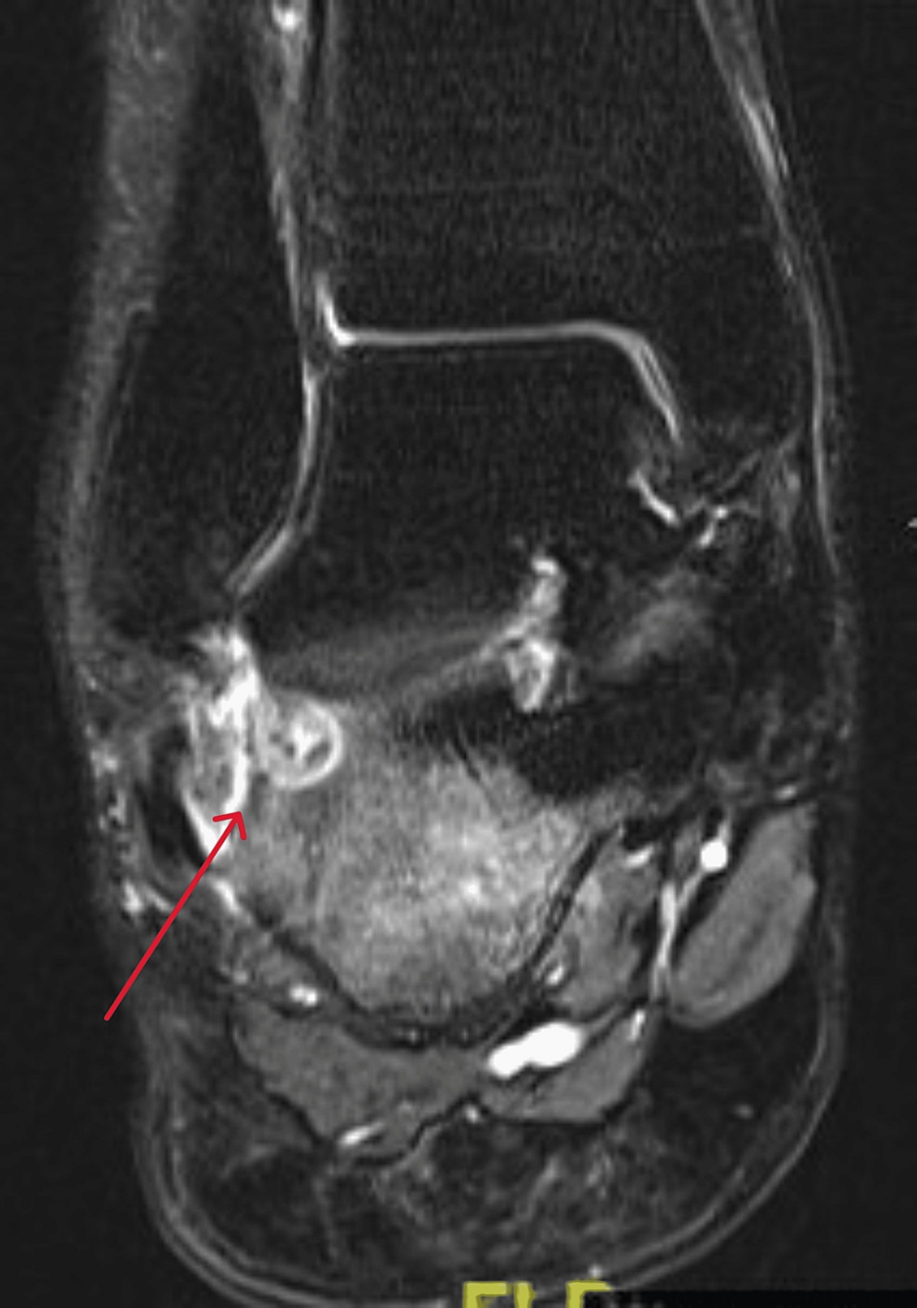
Coronal view MRI T2 window of the right ankle at the time of presentation MRI: magnetic resonance imaging
